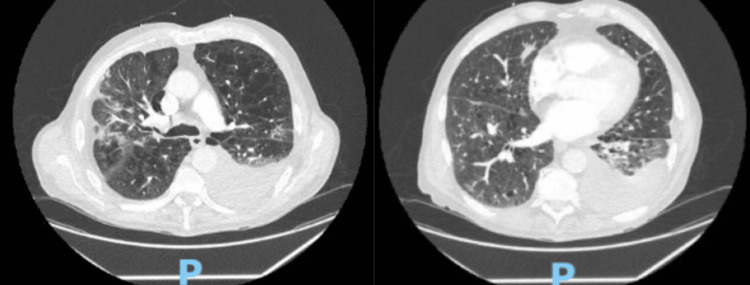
CT angiography of the Chest CT angiography of the chest showing severe emphysema, as well as bilateral patchy ground-glass opacities. Axial images demonstrate the ground-glass opacities most prominent in the right upper and left lower lobes. Pleural effusion noted on left side.
